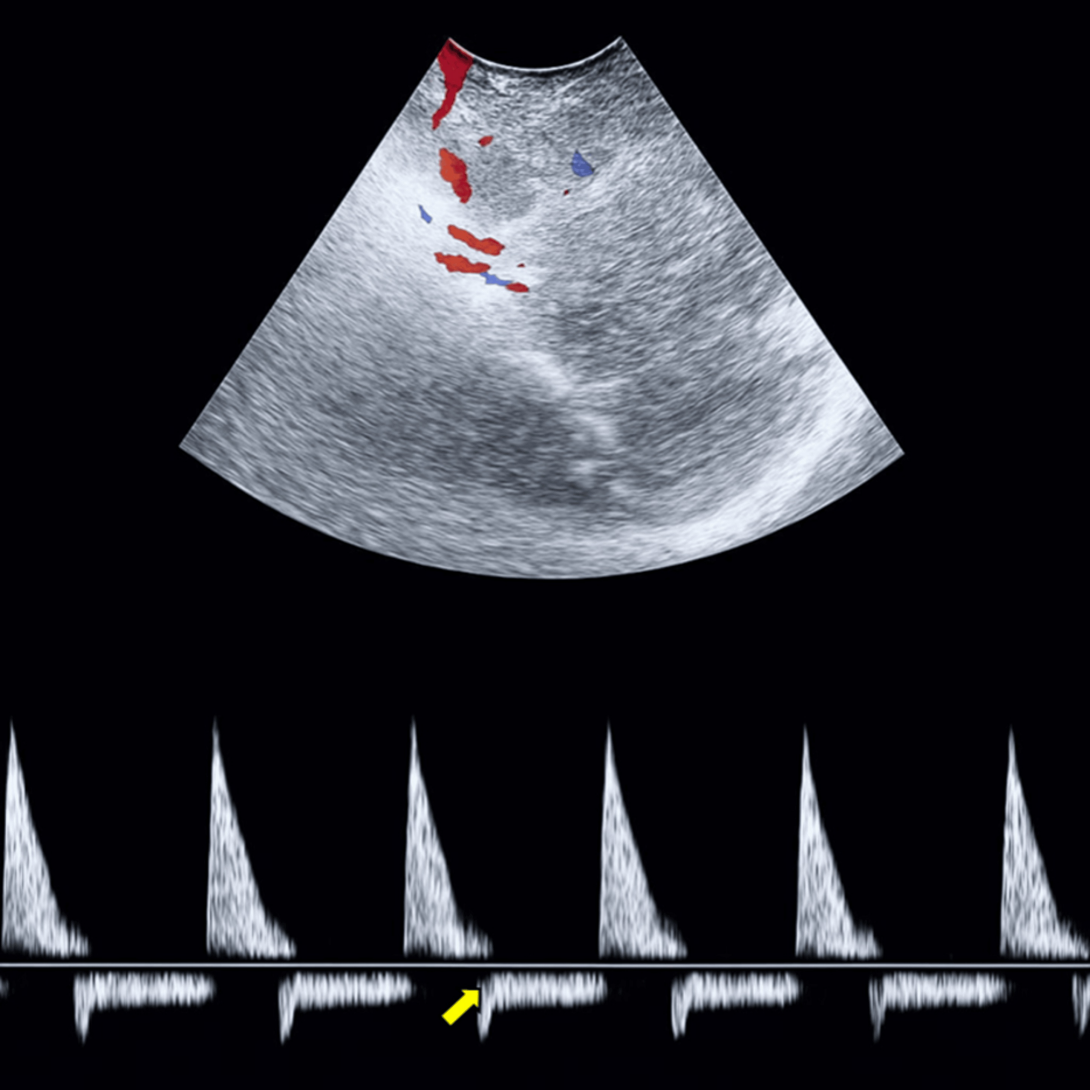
Transcranial Doppler of the right middle cerebral artery. A high-resistance pattern with reversed diastolic (arrow) flow is observed, suggestive of intracranial hypertension
